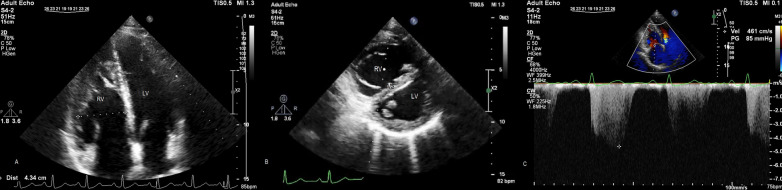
Echocardiogram showing right ventricular enlargement (A), septal flattening in systole and diastole in favor of right ventricular pressure overload (B), and high tricuspid regurgitation gradients (C). *RV* right ventricle, *LV* left ventricle, *IVS* inter-ventricular septum
